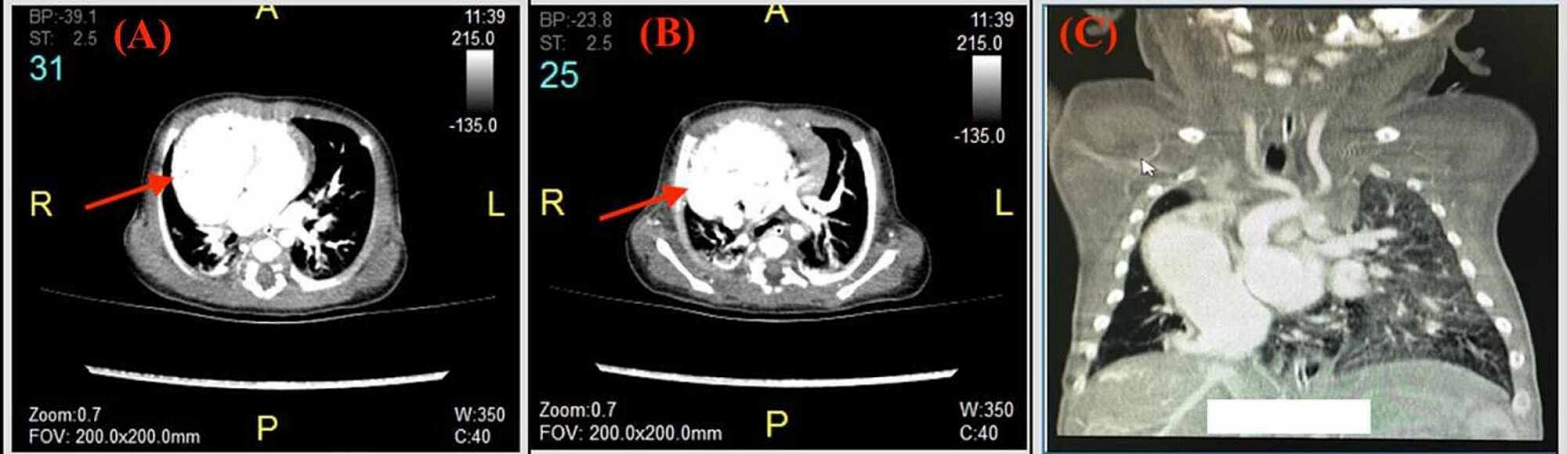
CT Chest (axial and coronal plane) Heart dextroposition and right pulmonary artery is smaller than left pulmonary artery
